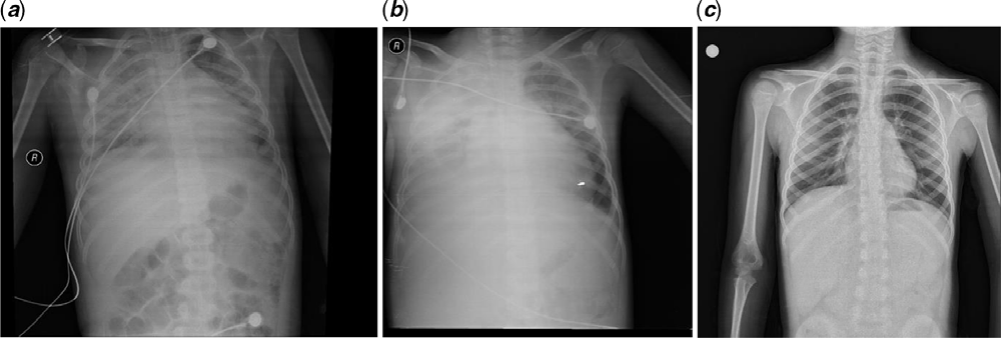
Chest X-ray radiographs (a posteroanterior radiograph of the chest in the upright position of the patient): from left to right. (*a*) admission to the hospital, (*b*) at the beginning of treatment, (*c*) after 14 days of treatment.
